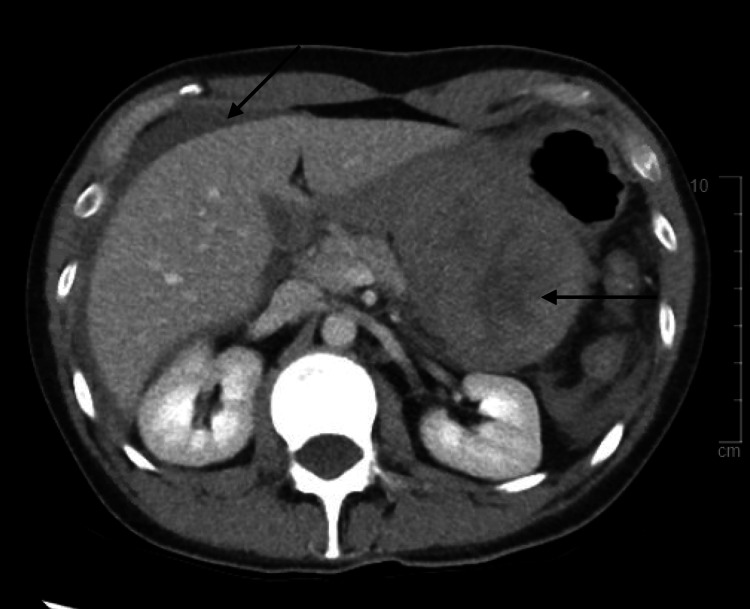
CT of the abdomen and pelvis with contrast performed at presentation showing an irregular large fluid collection upon the gastric body with a small volume of dense fluid.
